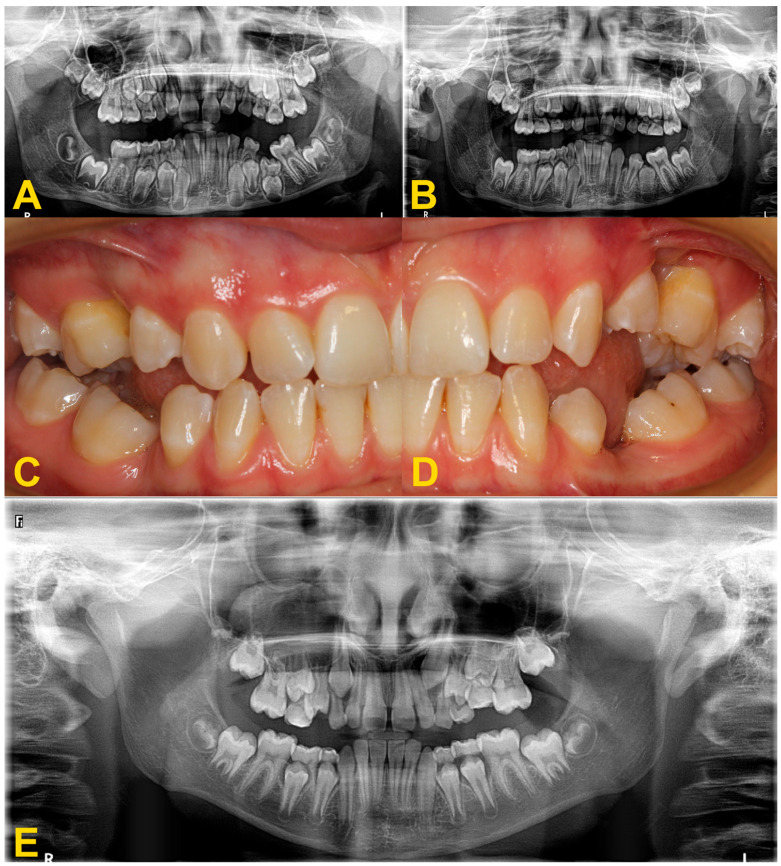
Disorders of the occlusal plane and displacement of the adjacent teeth: (A,B) panoramic X-rays—displacement of the adjacent teeth, bone defect and growth delay of the permanent successor due to the severe infraocclusion of tooth no. 75 before (A) and after the extraction of tooth no. 75 (B). Surgery was performed under general anesthesia with simultaneous extraction of teeth nos. 38 and 48 due to crowding. Lack of space for tooth no. 35, improvement of root development and position of tooth no. 35; (C,D) intraoral photography (another patient)—displacement of the adjacent teeth, disturbance of the occlusal plane with lateral open bite, and bone defect—situation after too-late removal of reincluded teeth with lack of permanent successors, (E) panoramic X-ray—moderate infraocclusion of tooth no. 65, displacement of the tooth no. 25 in relation to its antimere, incorrect position of the tooth no. 23 with suspected cyst, moderate infraocclusion of teeth nos. 74, 84, and 85, no resorption of the mesial root of tooth no. 85, mild infraocclusion of tooth no. 75.
